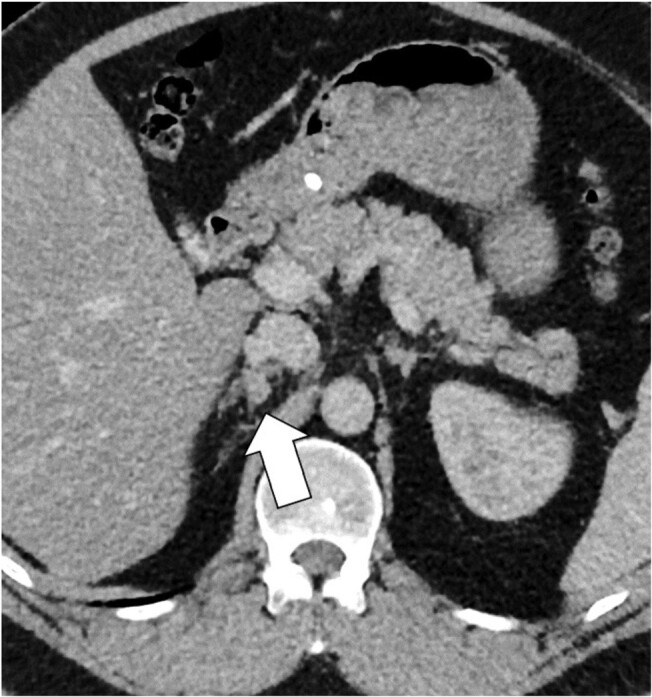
Noncontrast computed tomography image of the adrenal glands. Arrow indicates nodular thickening of the right medial limb.
